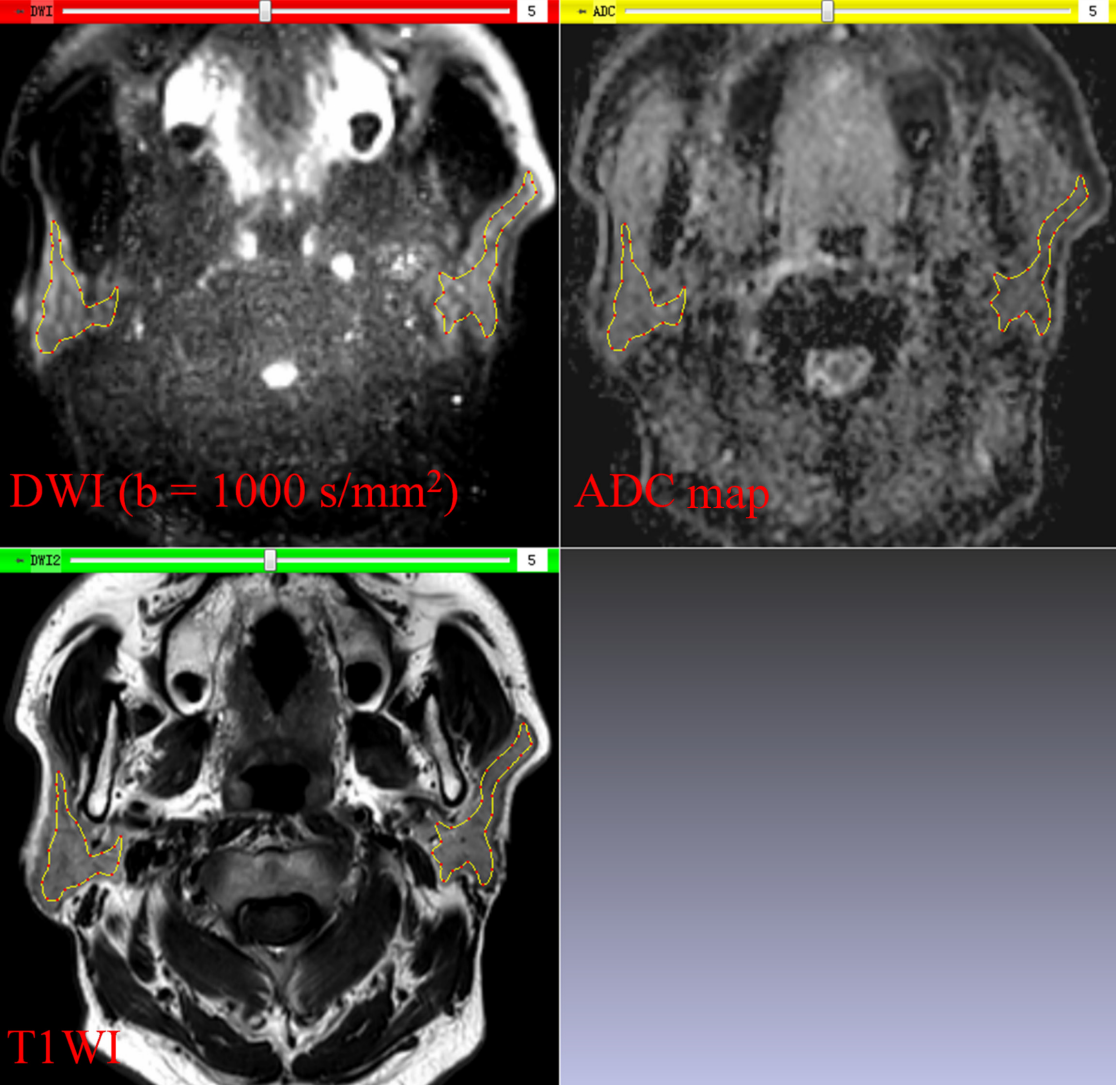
In-house software screenshot The region of interest is exactly copied on the diffusion weighted image (DWI) to the corresponding T1-weighted image (T1WI) and apparent diffusion coefficient (ADC) map in real time. Parotid glands can be distinguished from the adjacent structures on DWI and T1WI.
